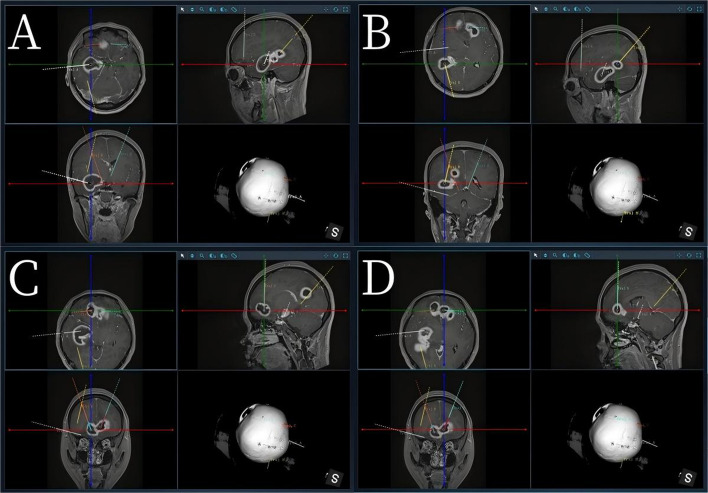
A–D Screenshot from Sinoplan software demonstrating trajectory planning based on the fusion of preoperative brain MR and CT images
